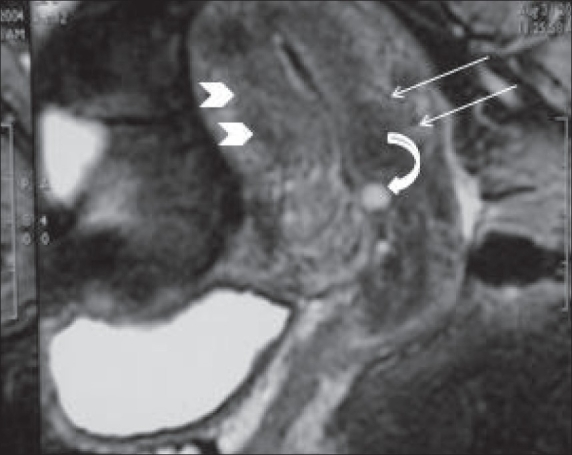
Sagittal T2W MRI of the uterus shows features of adenomyosis (more marked in the lower uterine segment) with thickened junctional zone of the myometrium (arrowheads) and a small hyperintense myometrial cyst (curved arrow)
